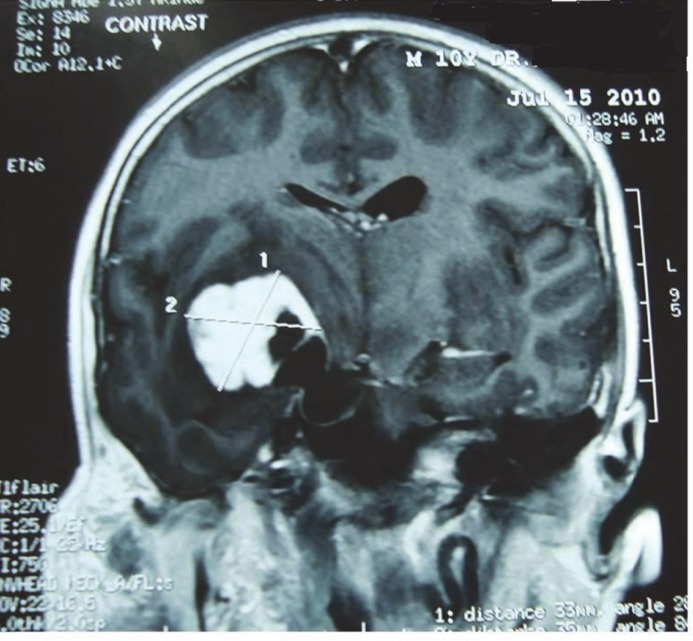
Coronal MRI scans of brain showing right parasellar mass
